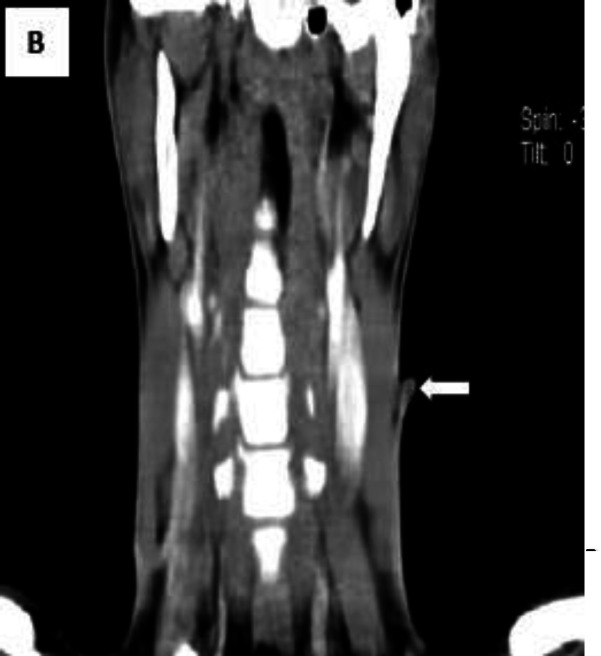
Spiral neck CT scan, coronal view. The sinus tract has been shown with an arrow as a low-density area just beneath the lesion
